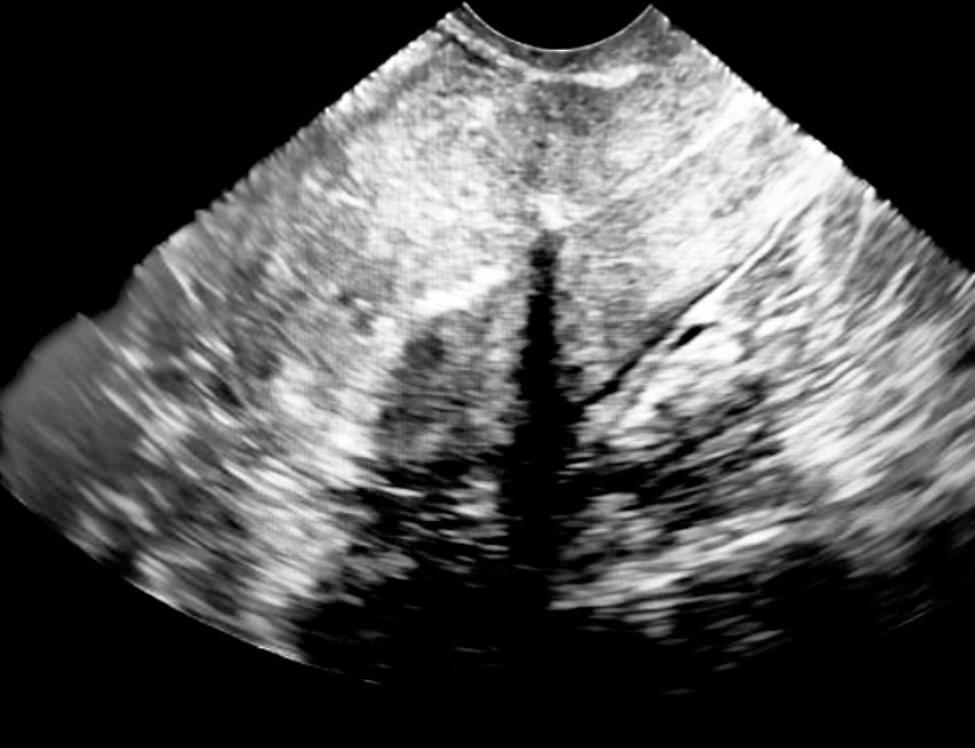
Transvaginal sagittal 2D ultrasound after evacuation of mass in the endometrial cavity by dilation and curettage. Ultrasound demonstrates resolution of the mass in the endometrial cavity
